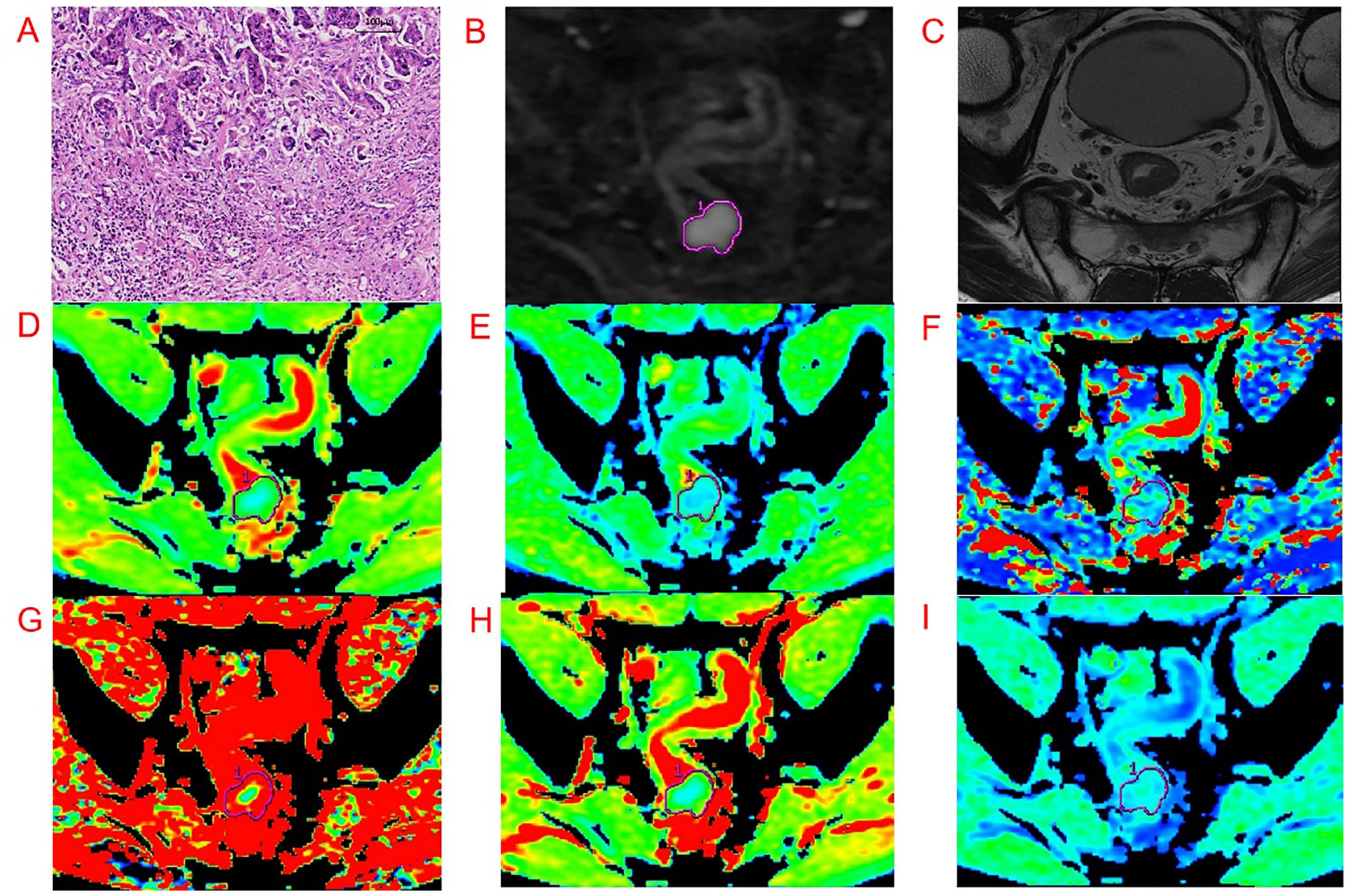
Representative images from an example 54-year-old male patient with RC with pathologically proven with Bd 3. Regions of interest were manually drawn along the border of the tumor on DWI, with a *b*-value of 1,000 s/mm^2^ and directly co-localized on all parametric maps. (A-I) are pathological imaging, DWI, oblique axial T2WI, ADC, *D*, *D, *f*, DDC, and α map, respectively.
